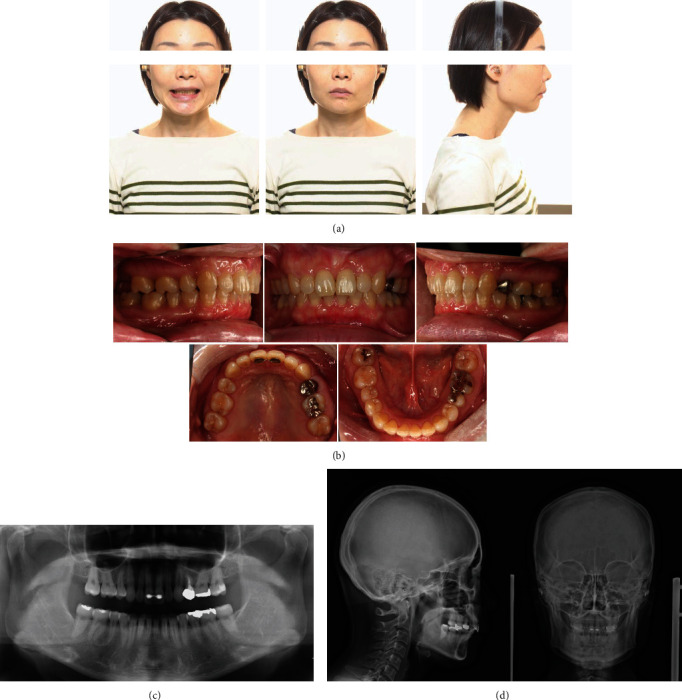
Two-year posttreatment records. (a) Extraoral photographs. (b) Intraoral photographs. (c) Orthopantomograms. (d) Posteroanterior and lateral cephalograms.
